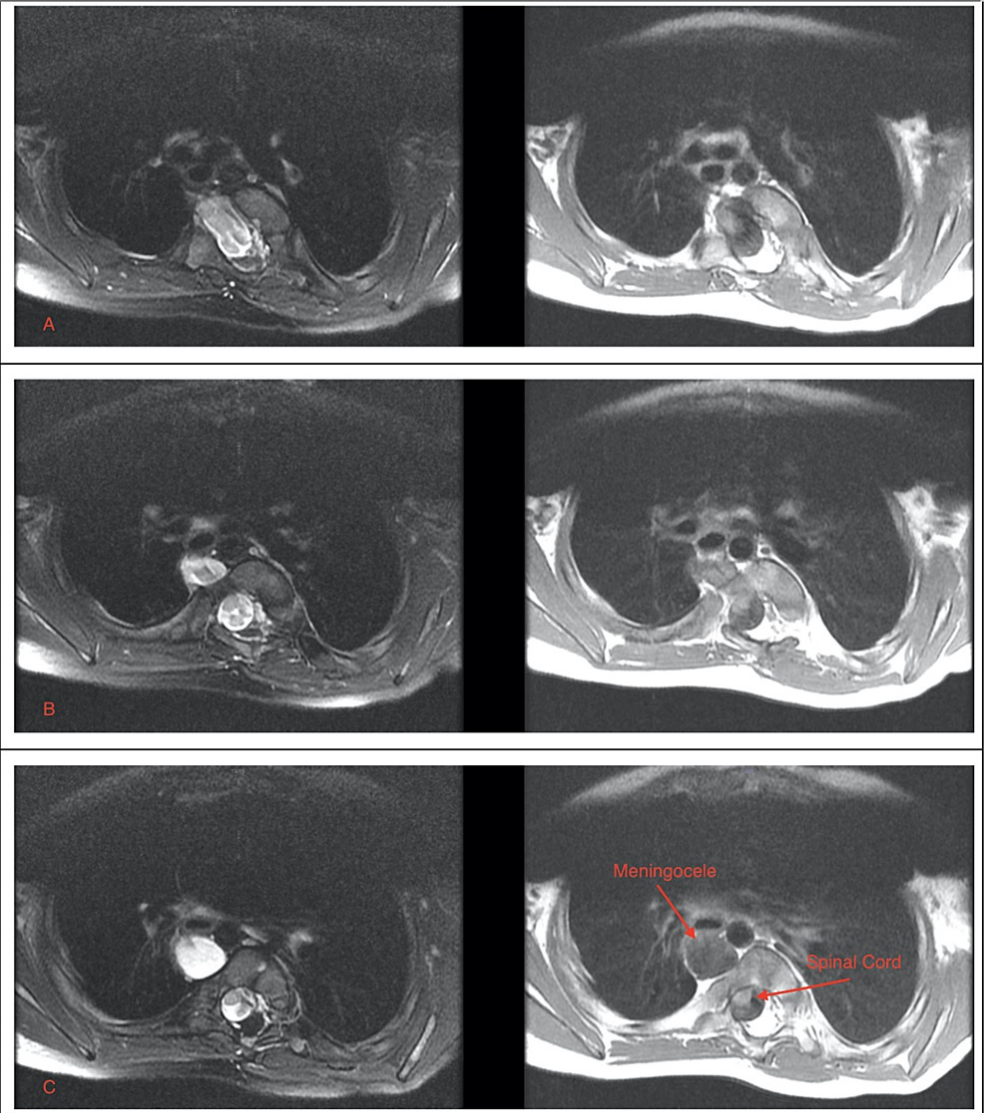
Selected axial T2-weighted and T1-weighted MRI of the thoracic spine showing the meningocele and tethered spinal cord in the vertebral column MRI: magnetic resonance imaging
